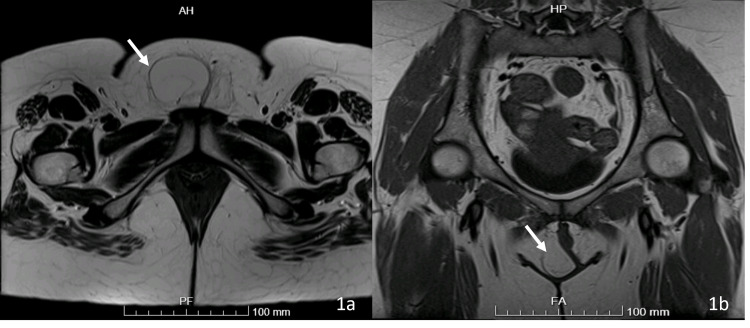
Axial (a) and coronal sections (b) of the magnetic resonance imaging of the pelvic floor.
